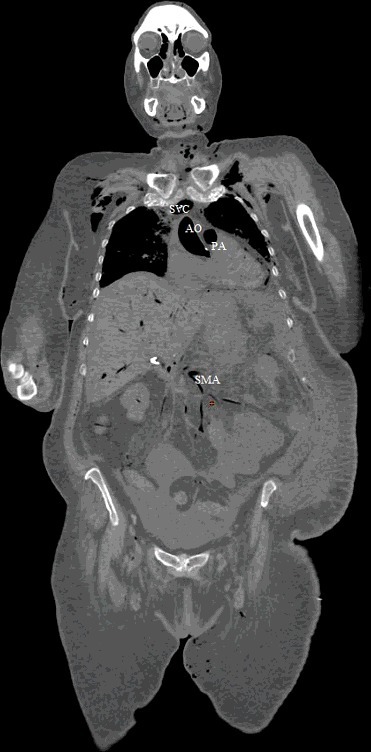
Coronal CT-soft tissue window: intravascular air in aorta (AO), pulmonary artery (PA), superior vena cava (SVC) and superior mesenteric artery (SMA). There is intravascular air in the liver and subcutaneous emphysema in the upper chest and neck soft tissues.
